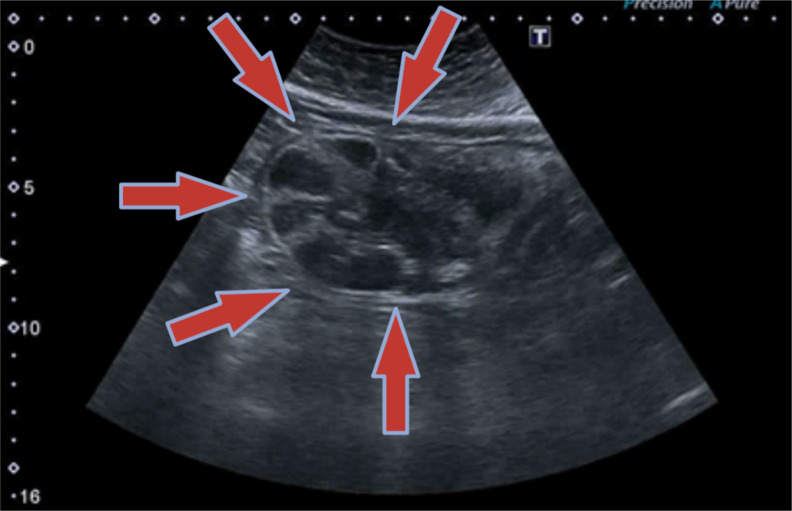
The membrane structure surrounding the small intestines in abdominal ultrasonography.
